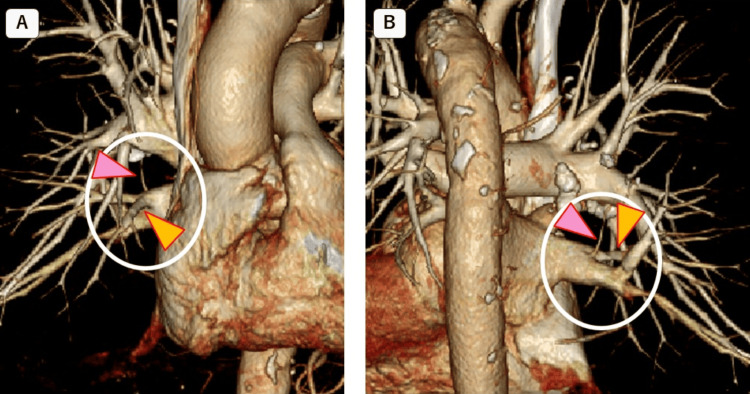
3D-MDCT before surgery (A) 3D-MDCT frontal view. (B) 3D-MDCT back view. The inferior pulmonary vein (PV) is shown in the white circle. The pink arrow shows the posterior segmental vein of the upper lobe (V^2^) and the yellow arrow shows the middle lobe PV; both PVs joining the inferior PV can be clearly seen 3D-MDCT: three-dimensional multidetector computed tomography
